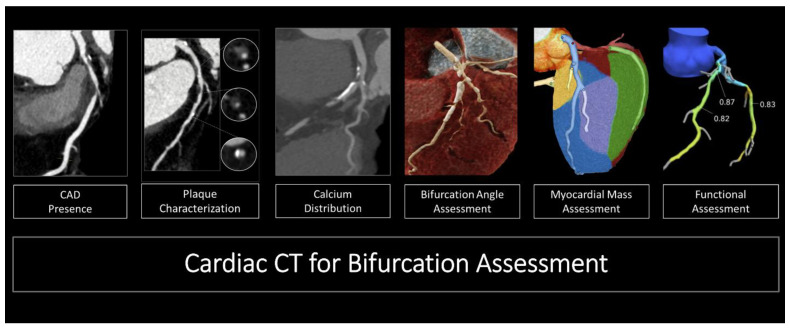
Application of Cardiac Computed Tomography Angiography for coronary bifurcation assessment. CAD—coronary artery disease; CT—computed tomography.
